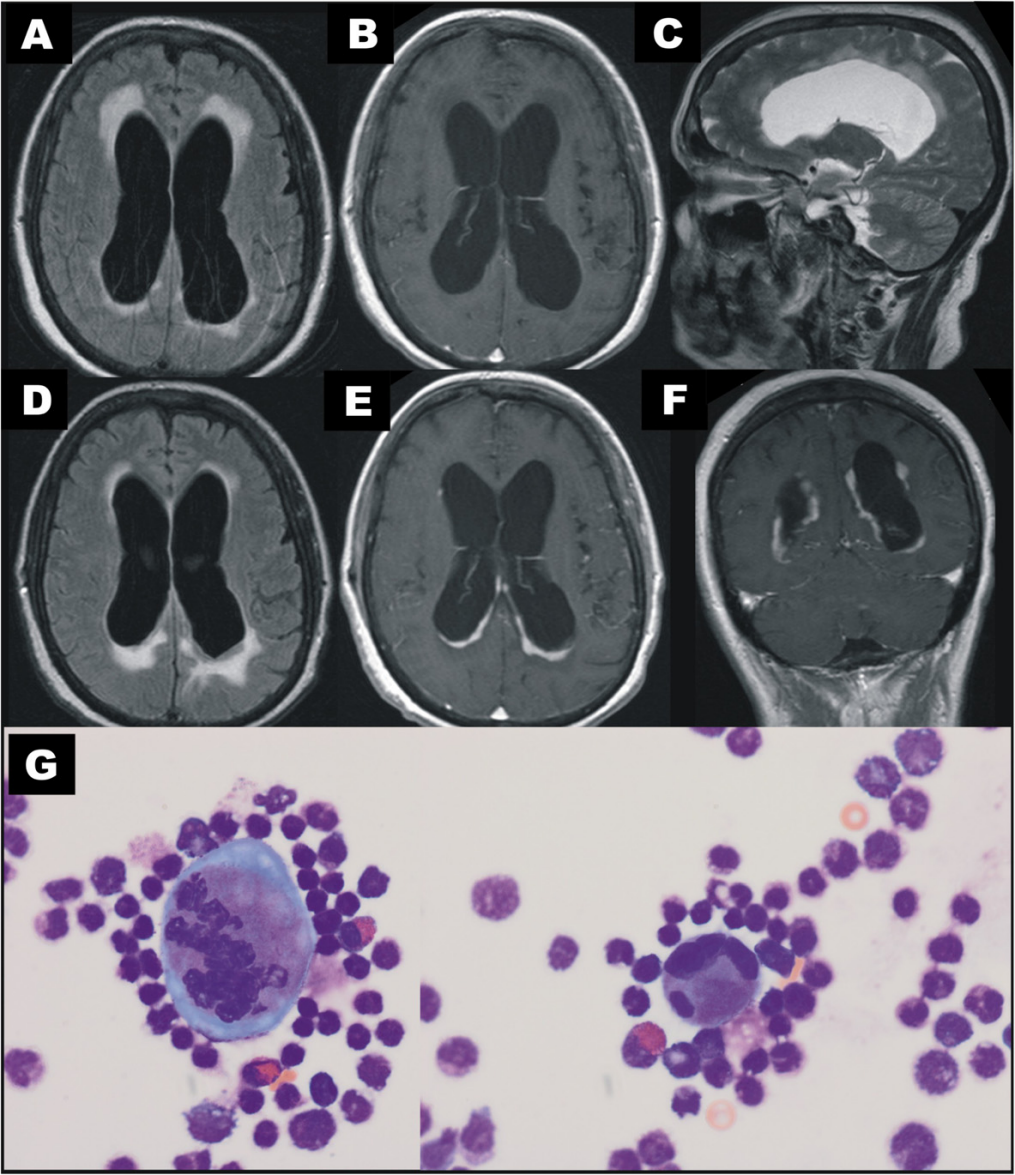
MR and CSF: First MR showed a hydrocephalic enlargement of the lateral ventricles (A FLAIR, B Gd-enhanced T1w, C T2w), while gadolinium-enhanced T1w did not show significant enhancement (B). Follow-up MR after secondary deterioration of the patient: showed leukemic infiltrations of the lateral ventricles walls (D FLAIR, E and F Gd enhanced T1w). CSF (G) revealed immature blasts with a pathological plasma-nucleus relation and basophilia of cytoplasm.
